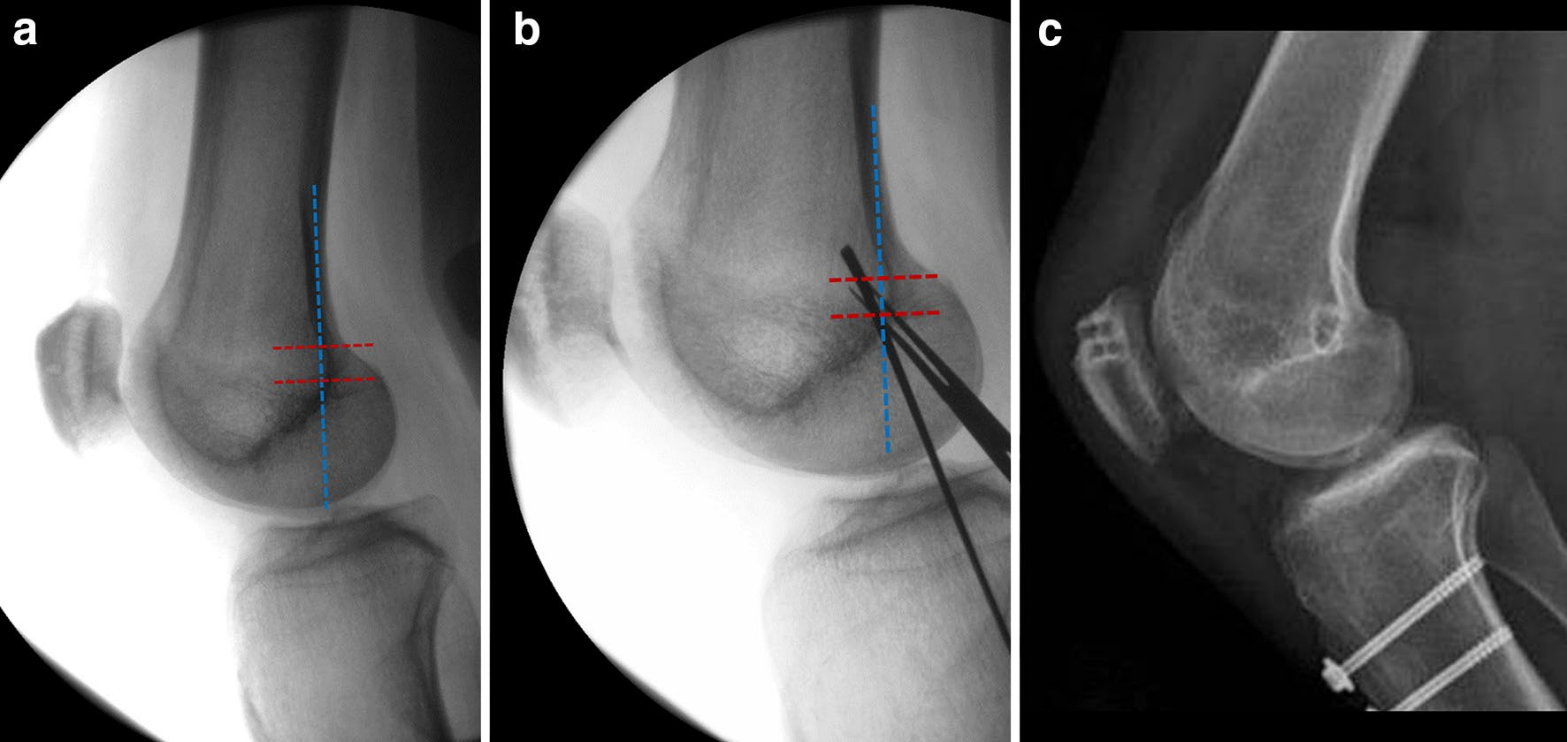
a Lateral radiograph of a knee with “Schöttle’s lines” in place. Radiographically, this is 1 mm anterior to the posterior femoral cortical line, 2.5 mm distal to the posterior origin of the medial femoral condyle, and proximal to the level of the posterior point of the Blumensaat line. b Lateral radiograph of the beath pin in place intraoperatively at the location of “Schöttle’s point.” c Correct position of femoral tunnel
